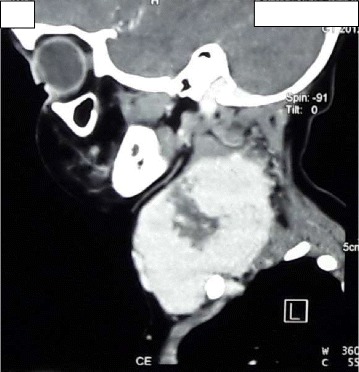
CT of neck (sagittal view)
